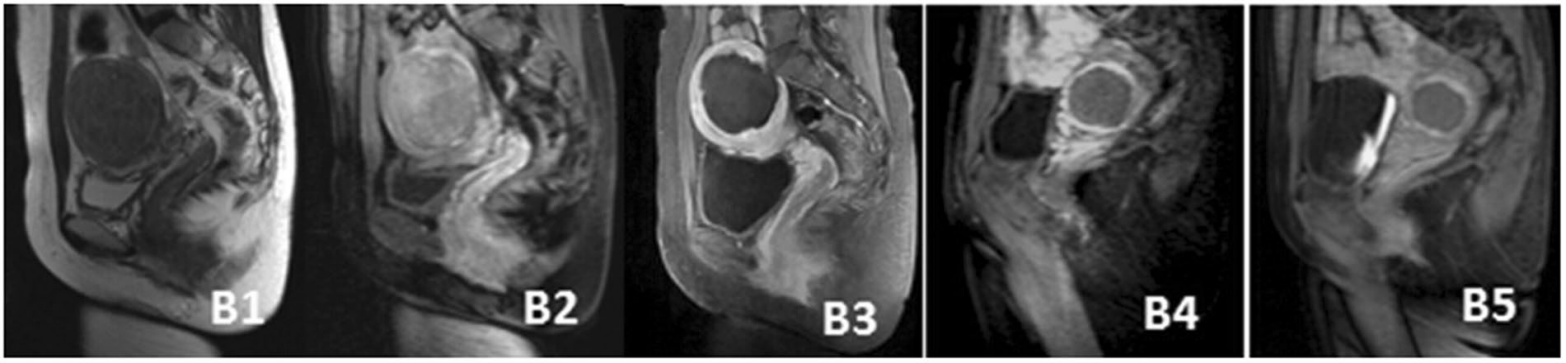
MRI of isointense fibroid before and after HIFU treatment: B1, T2WI-MRI before treatment; B2, fibroid shows enhancement on contrast-enhanced MRI before treatment; B3, 3 months after treatment; B4, 6 months after treatment; B5, 12 months after treatment.
